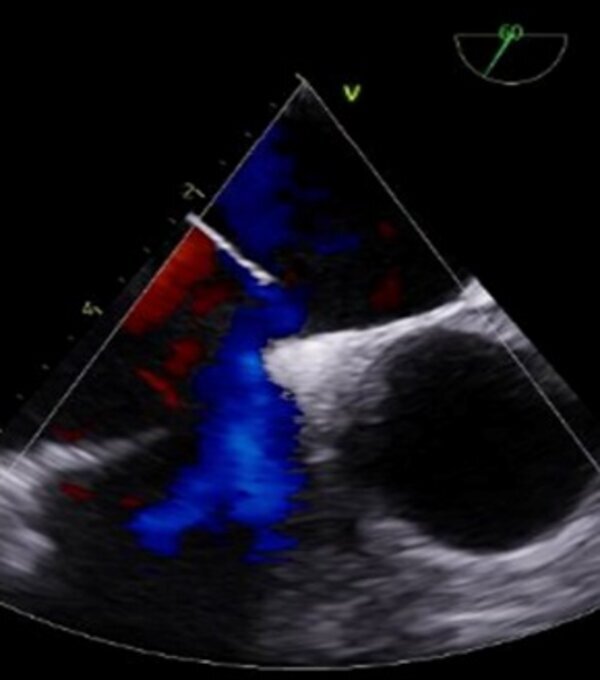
Transesophageal echocardiogram revealed persistence of an *ostium secundum* interatrial communication with a diameter of 7.5 mm, causing left-right shunt.
